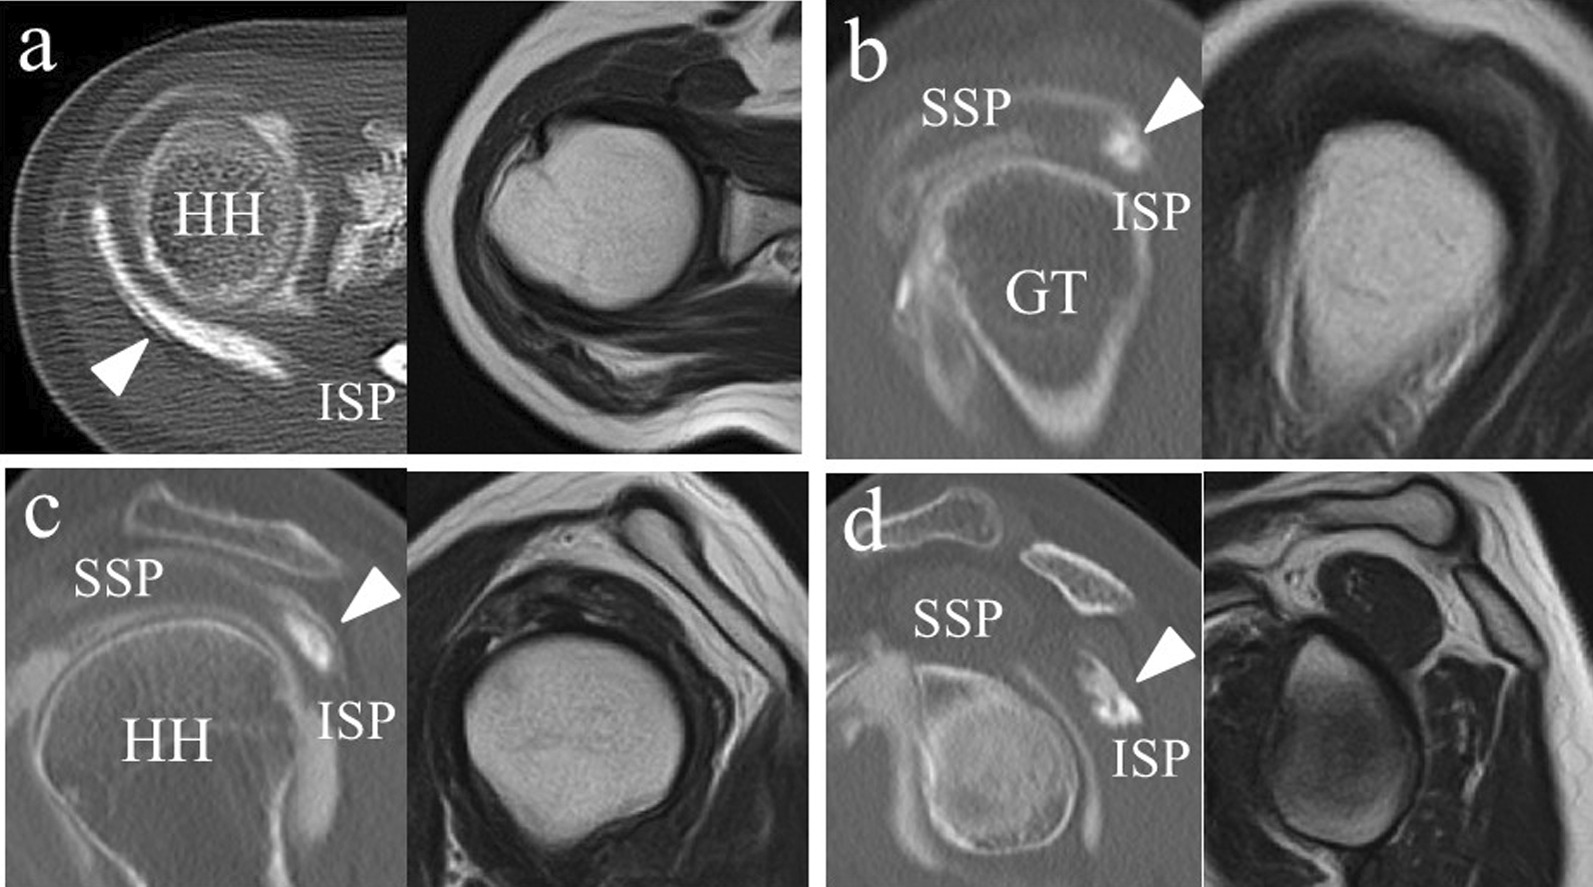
The infraspinatus tear is visualized by Computed tomography bursography. However, axial and sagittal T2-weighted MR images without fat suppression show a normal rotator cuff. a The axial image on Computed tomography bursography shows longitudinal pooling of contrast medium in the infraspinatus (arrowhead). b The sagittal image reveals that it communicates with the subacromial bursa near the insertion of the infraspinatus tendon (arrowhead) and (c, d) within the infraspinatus tendon and muscle body (arrowhead). *HH* head of the humerus, *GT* greater tubercle, *SSP* supraspinatus, *ISP* infraspinatus
